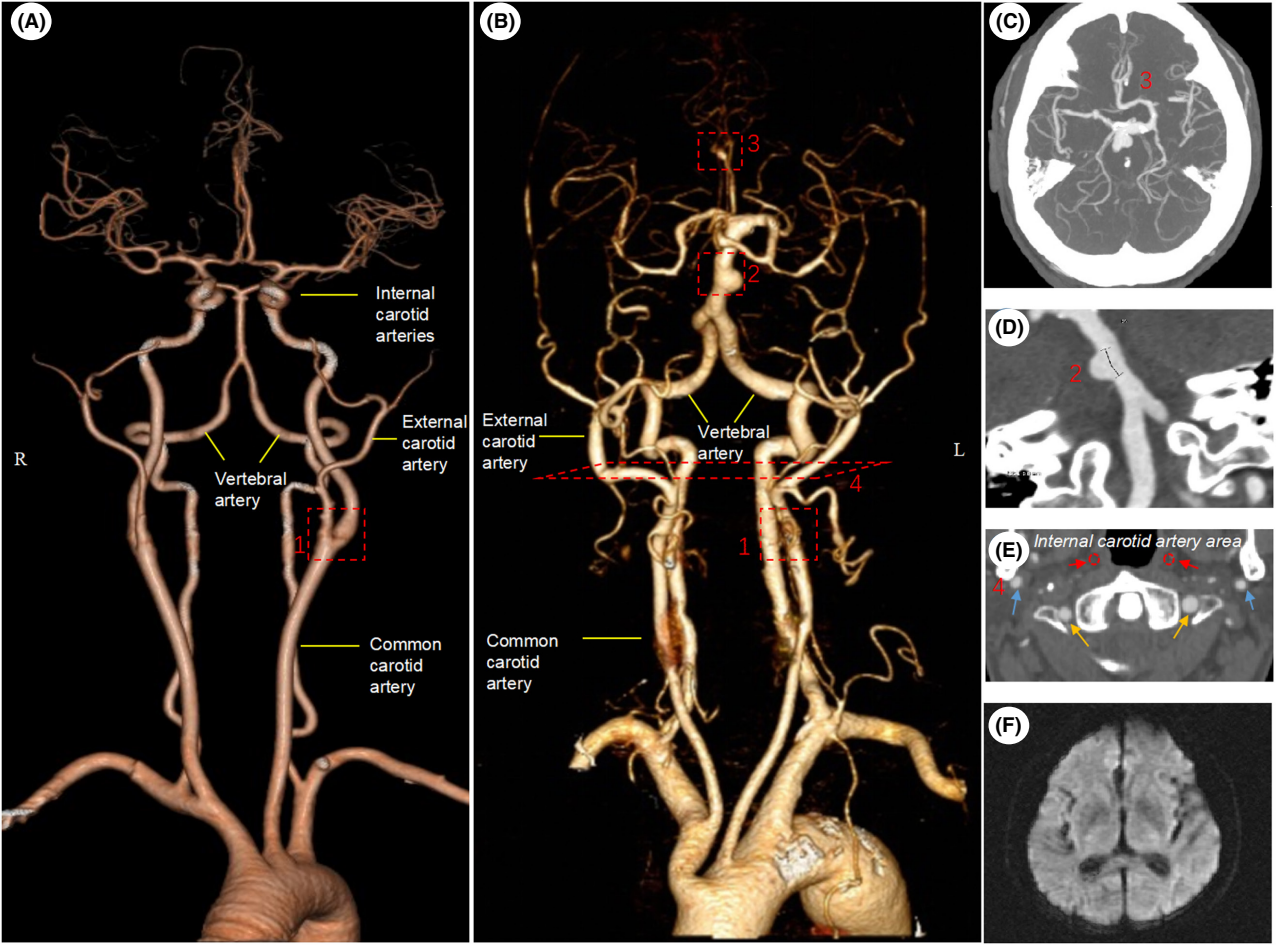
Absence of bilateral internal carotid arteries with multiple intracranial aneurysm but no acute infarction. (A) Three‐dimensional CTA assessment shown physiological anatomy of six large vessels supplying the brain, in which the common carotid artery is further divided into external carotid arteries (ECA) and ICA. (B) The patient had no ICA (Area 1) with enlarged vertebrobasilar arteries and ECA. Aneurysm appeared in basilar artery (Area 2) and left anterior cerebral artery A2 segment (Area 3). (C) Intracranial vascular reconstructed with maximum intensity projection (MIP) (D) Curvature plane reconstruction for basilar artery aneurysm (E) Axial location of bilateral ICA running area (red arrow, no imaging), external carotid artery (blue arrow) and vertebral artery (yellow arrow) as in panel B‐Area 4 dotted. (F) Diffusion weighted imaging (DWI) showed no acute cerebral infarction.
